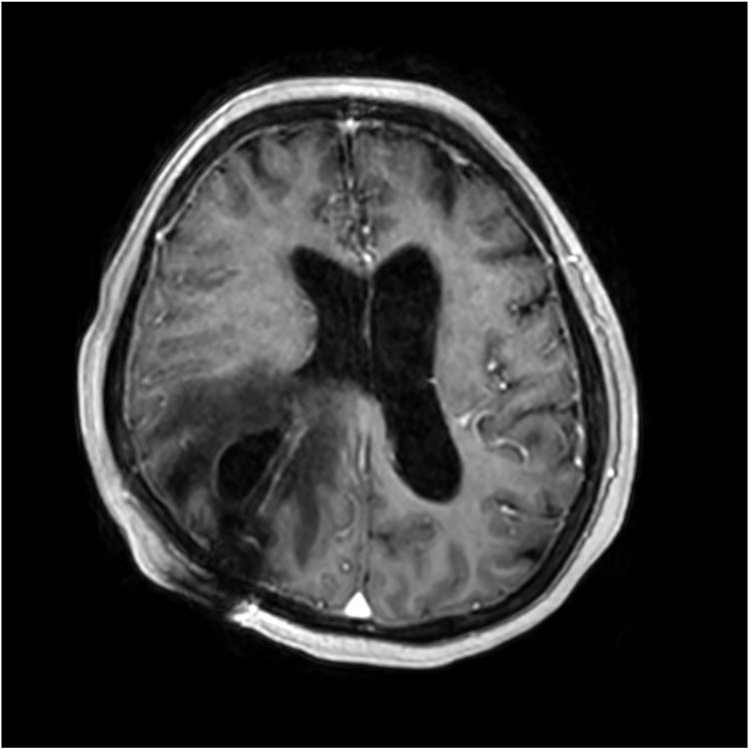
Contrast-enhanced T1-weighted MRI scan showing presence of the intraparenchymal cyst and surrounding vasogenic oedema.
